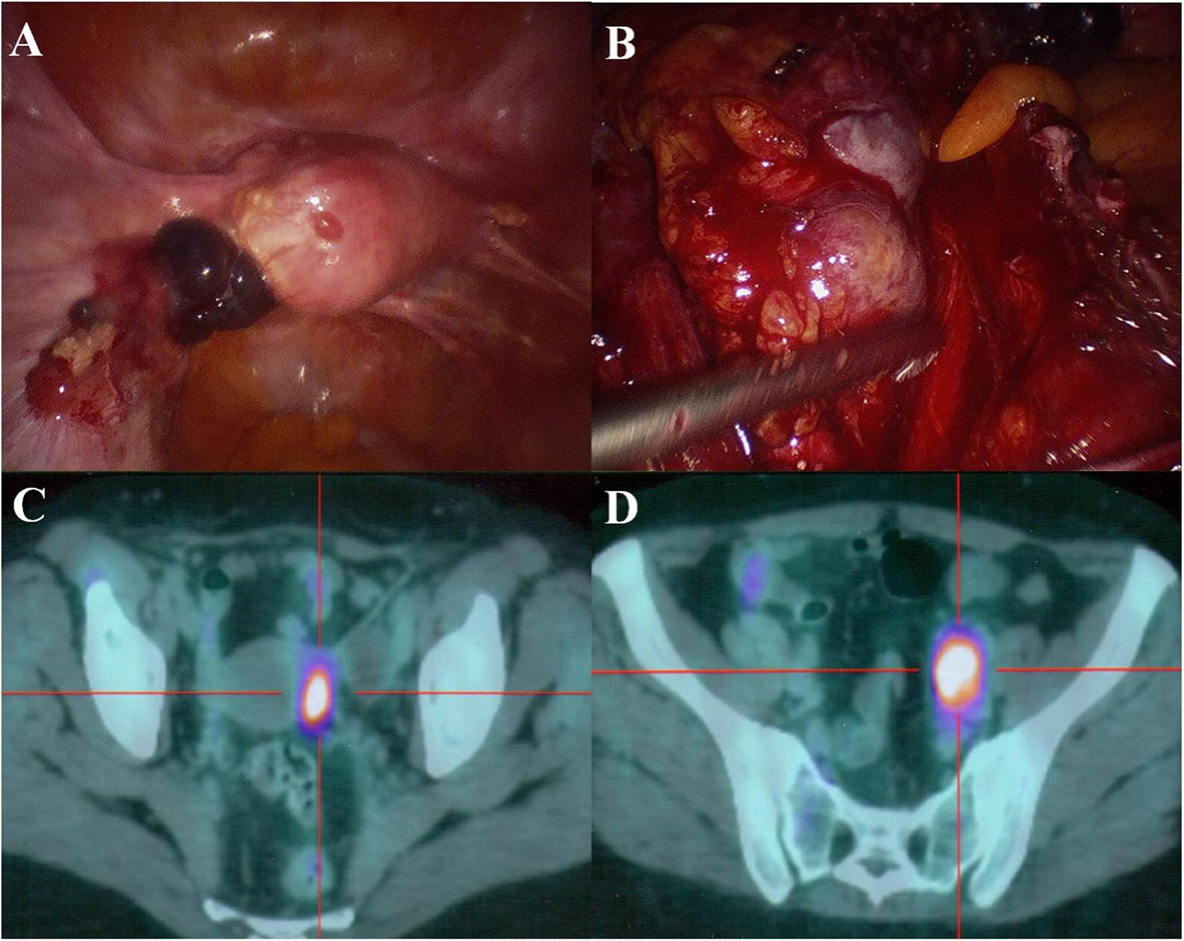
Laparoscopic and CT/PET characterization of the pelvic recurrence. A) Laparoscopic image of left pelvic peritoneal carcinomatosis. B) The large left pelvic lymphadenopathy. C) CT/PET image of a nodular mass near the uterus (SUV 10.6). D) Left pelvic lymph nodes (SUV 10.8).
